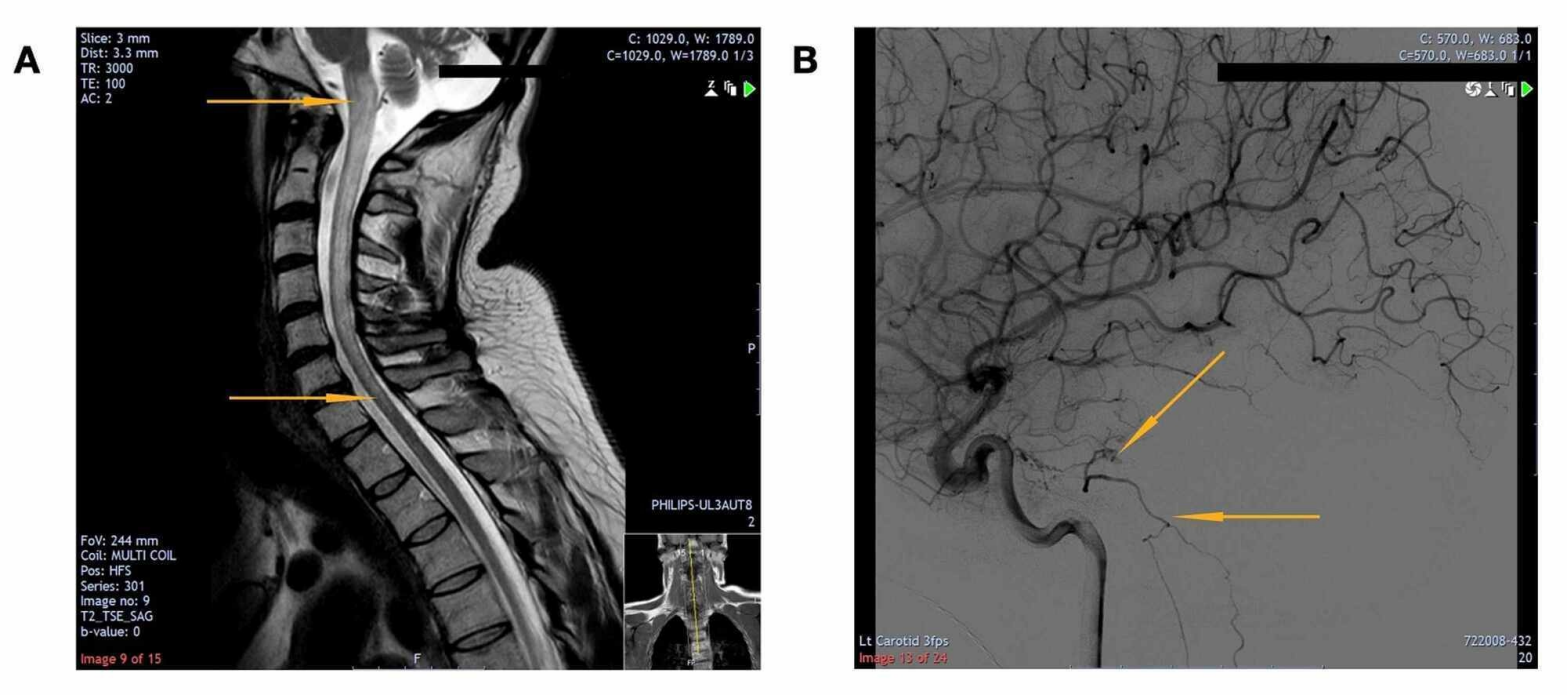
(A) Sagittal T2-weighted MRI scan showing worsening signal changes (orange arrows) and (B) DSA showing recurrence of the previously treated dural AVF (orange arrows) MRI (magnetic resonance imaging), DSA (digital subtraction angiography), AVF (arteriovenous fistula)
